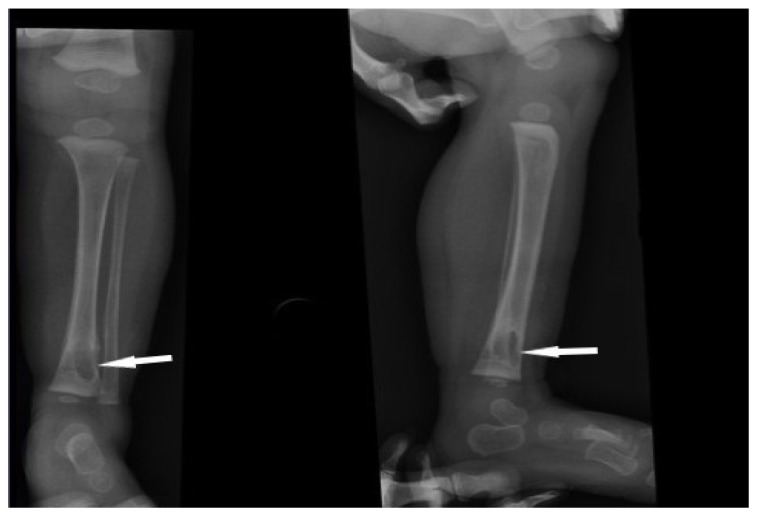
Radiographic image of bone osteolytic changes within the distal metaphysis of the tibia (white arrows)—at the time of diagnosis.
